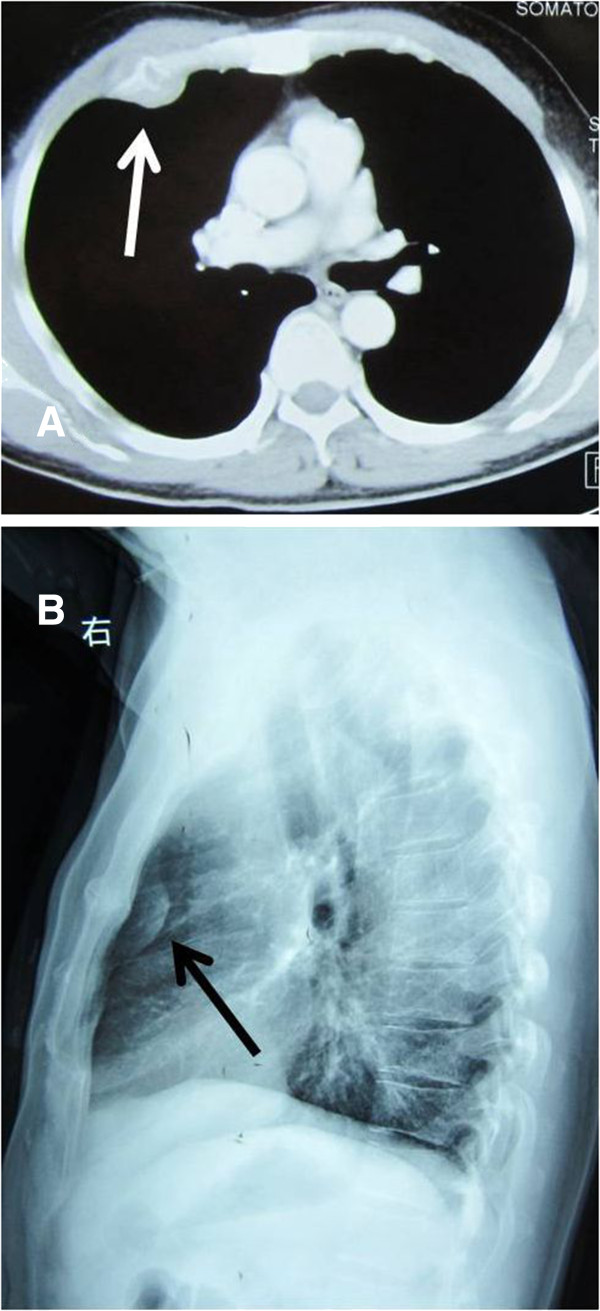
Chest X-ray and CT showed a mass on the right side of the chest wall. A and B: Chest X-ray and contrast-enhanced CT revealed a well-defined soft tissue mass (arrowhead) on right side abutting the chest wall, with invasion into the third rib.
